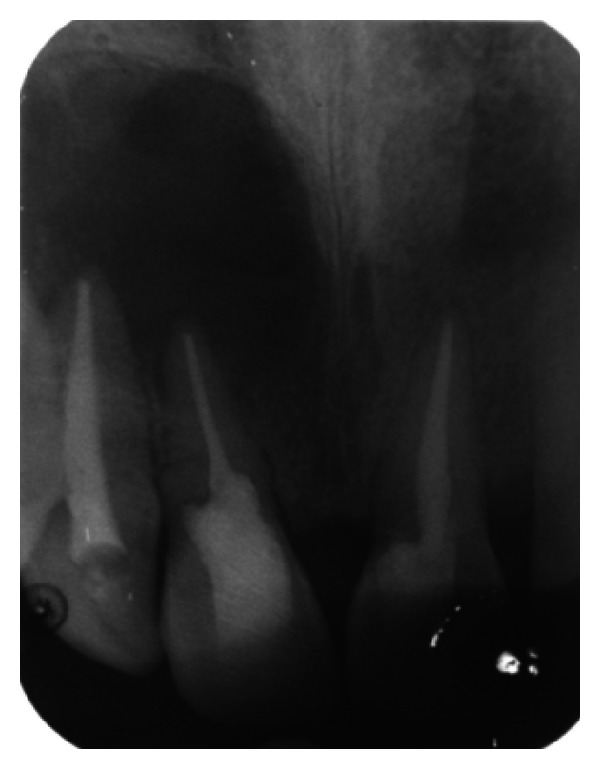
1-month followup IOPA radiograph.
